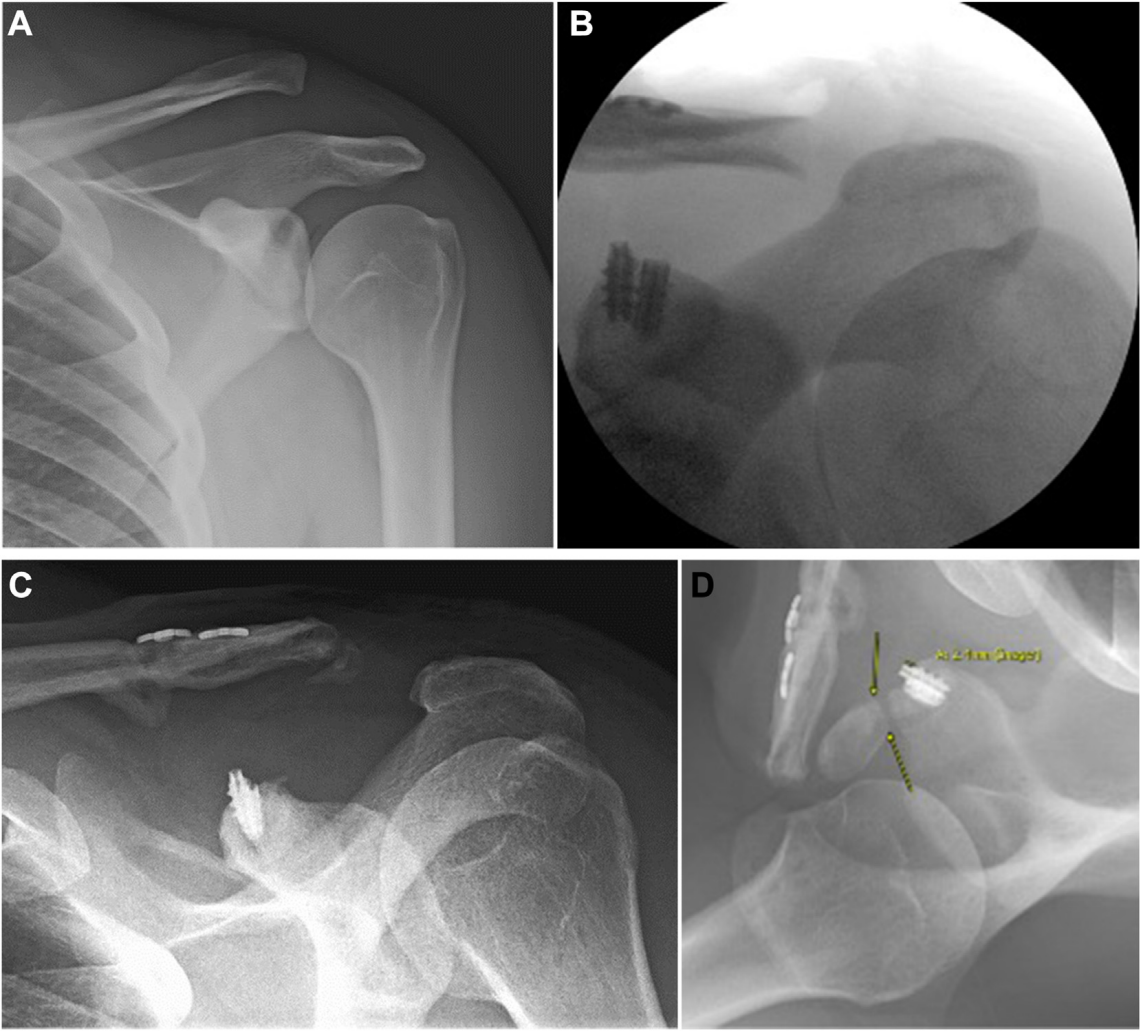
(A) Preoperative X-ray imaging (Zanca^20^ view) demonstrating Rockwood type 5 ACJ dislocation. (B) Intraoperative fluoroscopic imaging showing ACJ reduction with suture anchors fixation. (C) Three weeks postoperative X-ray imaging (Zanca^20^ view) demonstrating loosening of suture anchors and partial loss of reduction of the ACJ. (D) Two years postoperative X-ray imaging (Axillary view) demonstrating a coracoid fracture distal to the suture anchors. *ACJ*, acromioclavicular joint.
